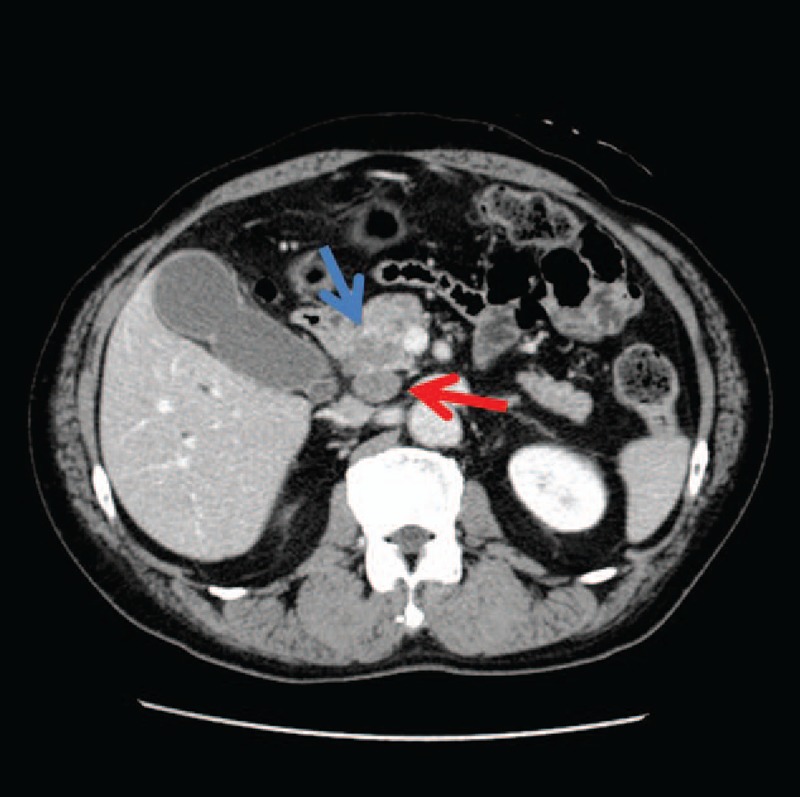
Contrast-enhanced computed tomography images showing a tumor in the pancreatic head measuring 3.0 × 2.5 cm in diameter and invading the common bile duct (indicated by a blue arrow). Lymphadenopathy was observed posterior to the pancreatic head (red arrow).
